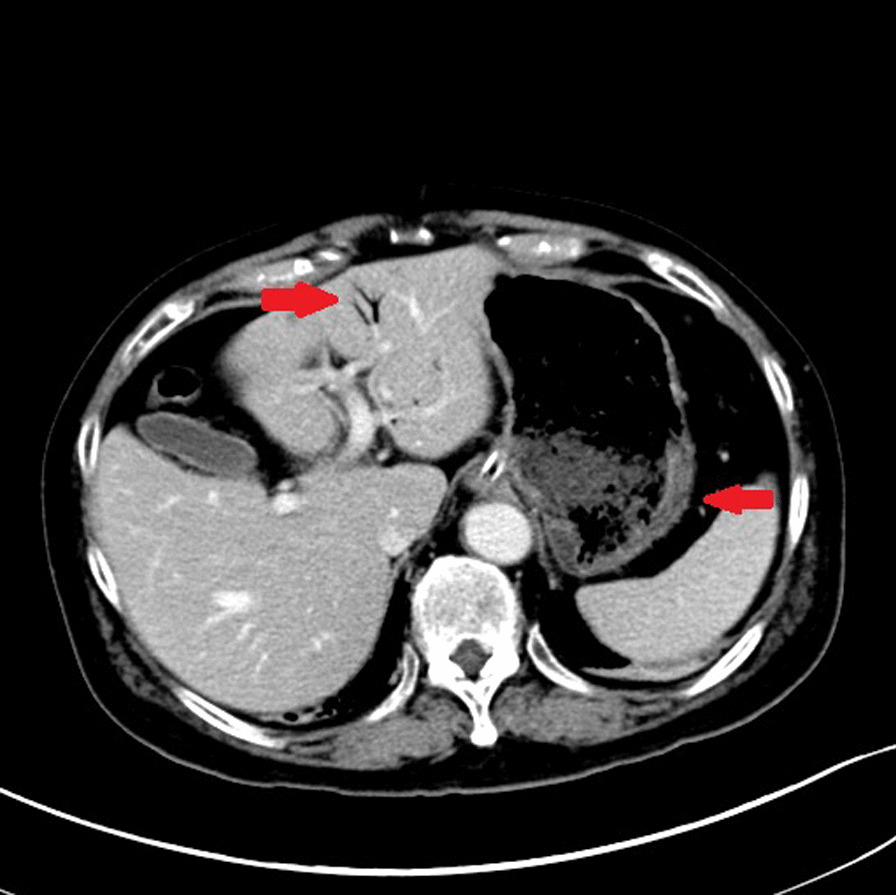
The computed tomography of the abdomen demonstrated venous air in portal veins, thickened gastric fundus wall with gastric pneumatosis
